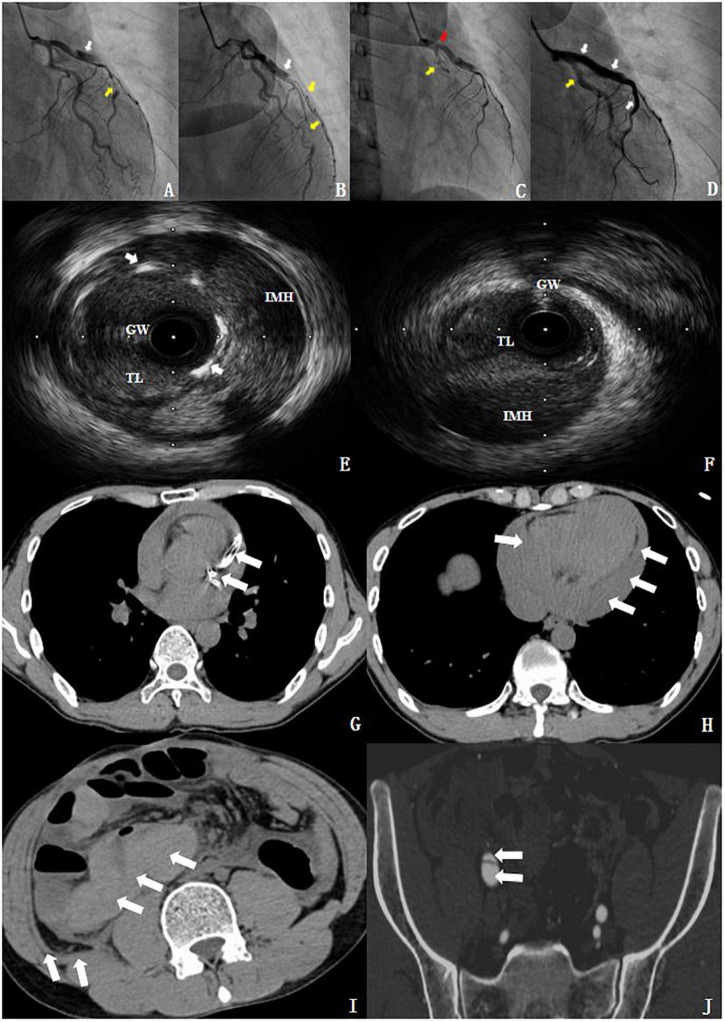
Imaging findings. (A,B) Coronary angiography (CAG). The white arrow shows a type 1 dissection at the proximal segments of LAD. The yellow arrow indicated a type 2 dissection at the middle segments of the LAD and the diagonal branch. (C) The patient showed progression of coronary artery dissection during the CAG. The red arrow demonstrates that the dissection involved the LM and the yellow arrow indicates a type 4 dissection of the LCX. (D) After revascularization. The white arrow shows the TIMI flow grade 3 at the LM and LAD. The yellow arrow indicates the TIMI flow grade 3 at the LCX. (E) IVUS showed a massive IMH from the media external to the LAD stent; the white arrow indicated the stent struts. (F) The true lumen (TL) of the median LCX segment was severely deformed by the dissection and the massive media layer hematoma. GW, guidewire. (G,H) The chest CT revealed a stent in the left anterior descending coronary artery and hemopericardium. (I,J) The abdominal and pelvic CT showed intestinal wall swelling, hematocele, retroperitoneal hematocele, and an iliac vascular dissection (see section “Supplementary material”).
